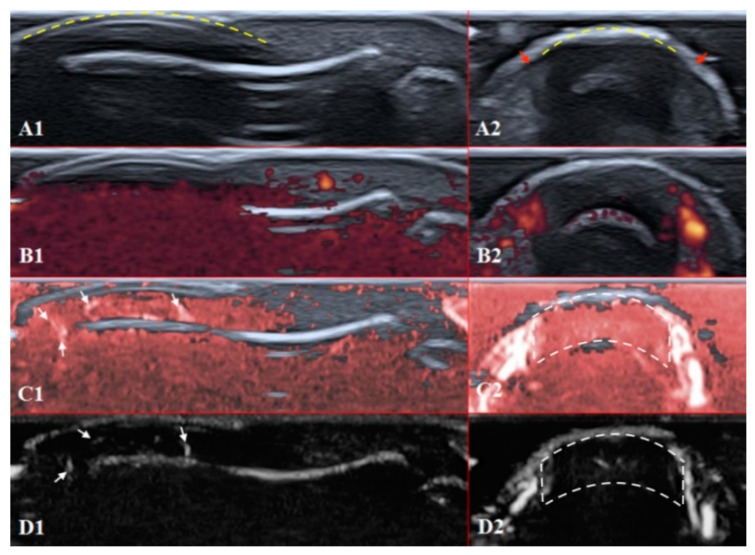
Ultrasound images of the nailfold of the fifth finger of the patient with a late course of SSc: (A1,A2) B mode/grayscale; yellow dotted lines mark the nail, red arrows mark the lateral nailfold, which is decreased due to sclerodactyly. (B1,B2) PD; there is an avascular zone in B2 in the deeper layers of the nailfold; B1 does not show any vascular sign with maximal gain. (C1,C2) cSMI; white arrows mark the true flow in the random noise background; the area marked by dotted lines in C2 is also avascular and the true flow signals on the sides show the lateral nailfold. (D1,D2) mSMI; confirms cSMI findings.
